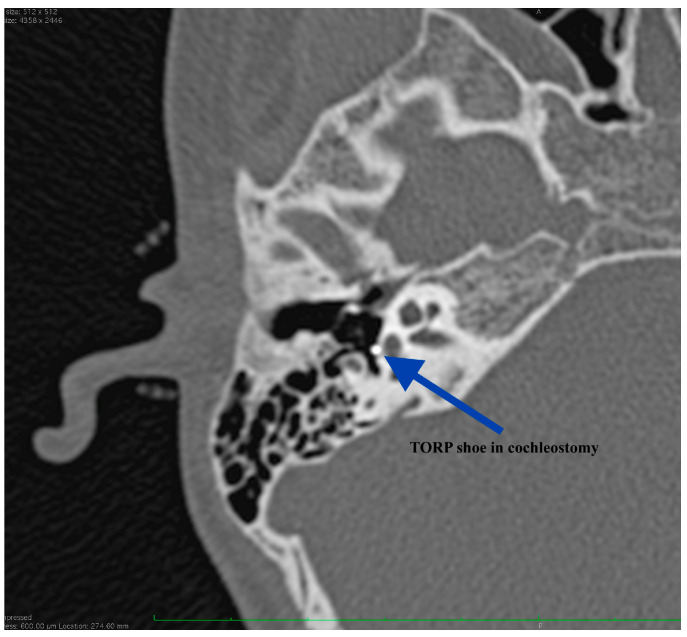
CT scan at 9 months after surgery, showing proper positioning of the prosthesis: TORP shoe in the cochleostomy.
